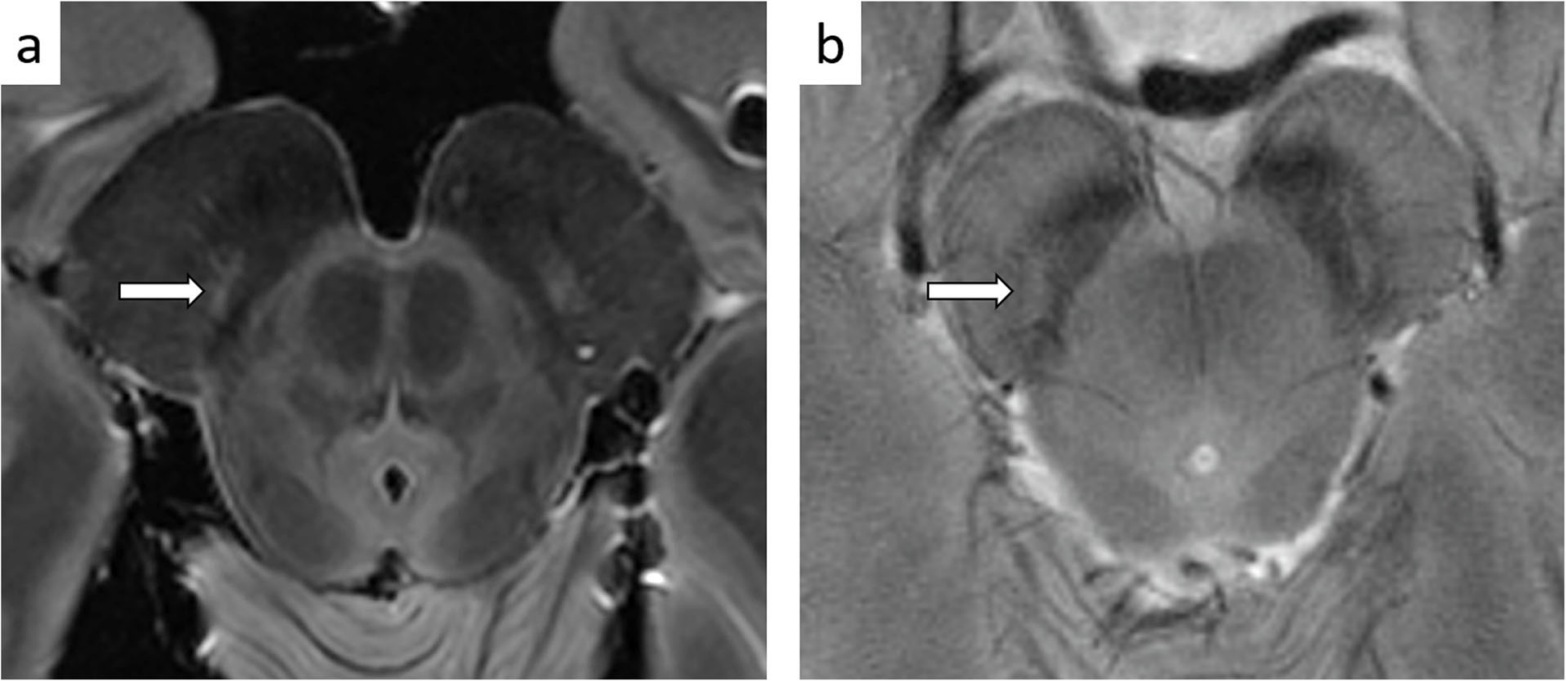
Ex vivo* and *in vivo* MR imaging of the SN. The oval-shaped hyperintense formation indicated by arrows corresponds to nigrosome 1 (N1). a Proton density axial image of an *ex-vivo* sample. b Gradient echo imaging at high resolution allows to define the N1 formation with typical “swallow tail appearance” in healthy subjects *in vivo*
